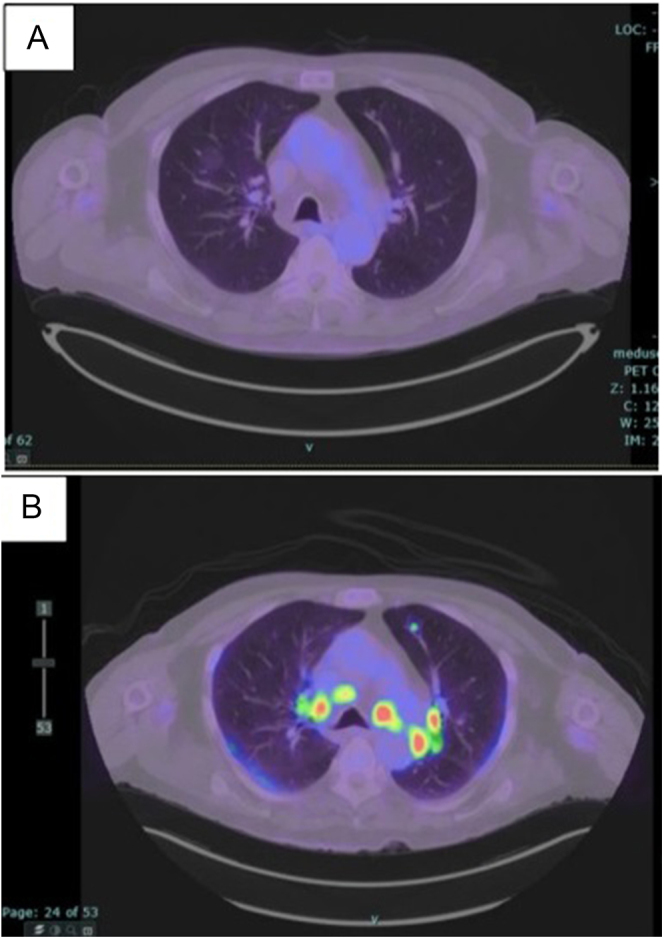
Axial CT/PET (A) before immunotherapy and (B) after immunotherapy.
